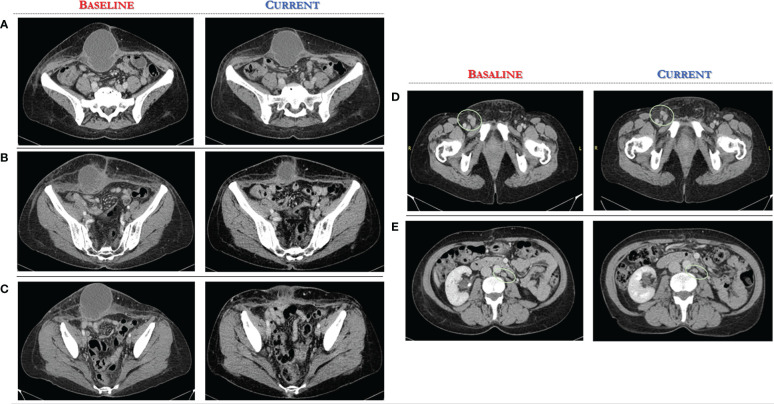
Computed Tomography scan demonstrates significant reduction of target lesions after 4 months of Alpelisib treatment compared with the corresponding pre-treatment scan. (A-C) axial CT scan, several sections of peritoneal carcinomatosis pre and post alpelisib treatment. (D, E) axial CT scan, several sections of secondary lymph nodes in the right inguinal and paraaortic region pre and post alpelisib treatment.
